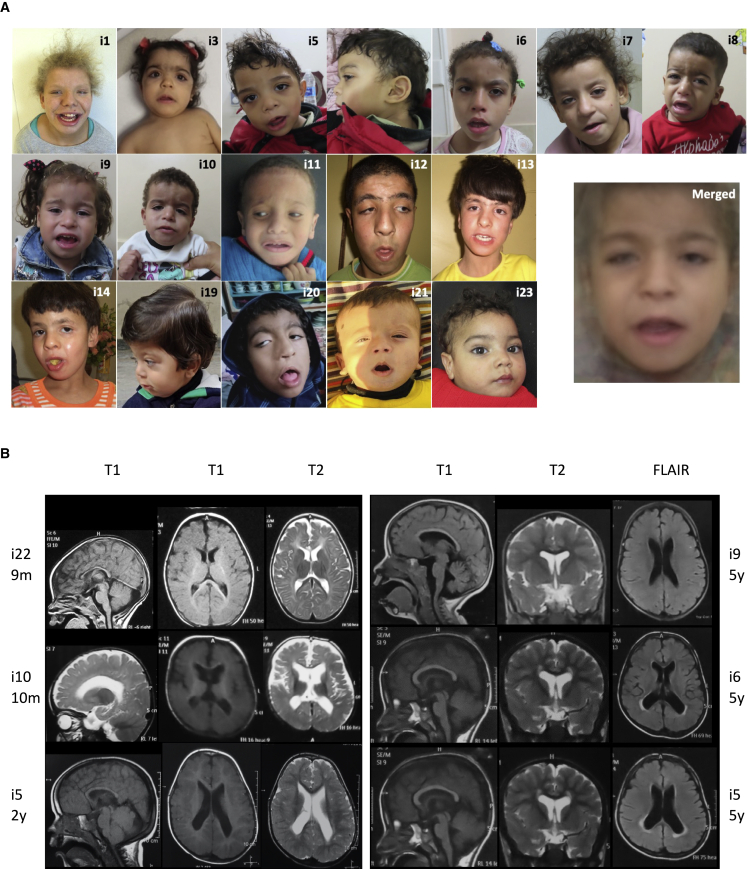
Photos of the affected individuals included in the cohort and brain MRI results (A) Affected individuals showed consistent coarse facial features. A merged image was obtained by the Facer tool. (B) Brain MRI of individuals with *TMEM147* disease-causing variants. Thin corpus callosum is present in all individuals whose brain MRIs were available. Subcortical atrophy with ventricle enlargement is observed in all individuals except i22 (F15-V-1) and i9 (F6-V-6) and was more pronounced in i10 (F6-V-7) at 10 months of age, associated with a cortical atrophy. According to age, myelination remains poor on MRI performed at 5 years of age (i5 – F4-IV-9, i6 – F5-IV-2, and i9 – F6-V-6), particularly in the temporal white matter with a hypersignal flair of the periventricular white matter in two individuals.
